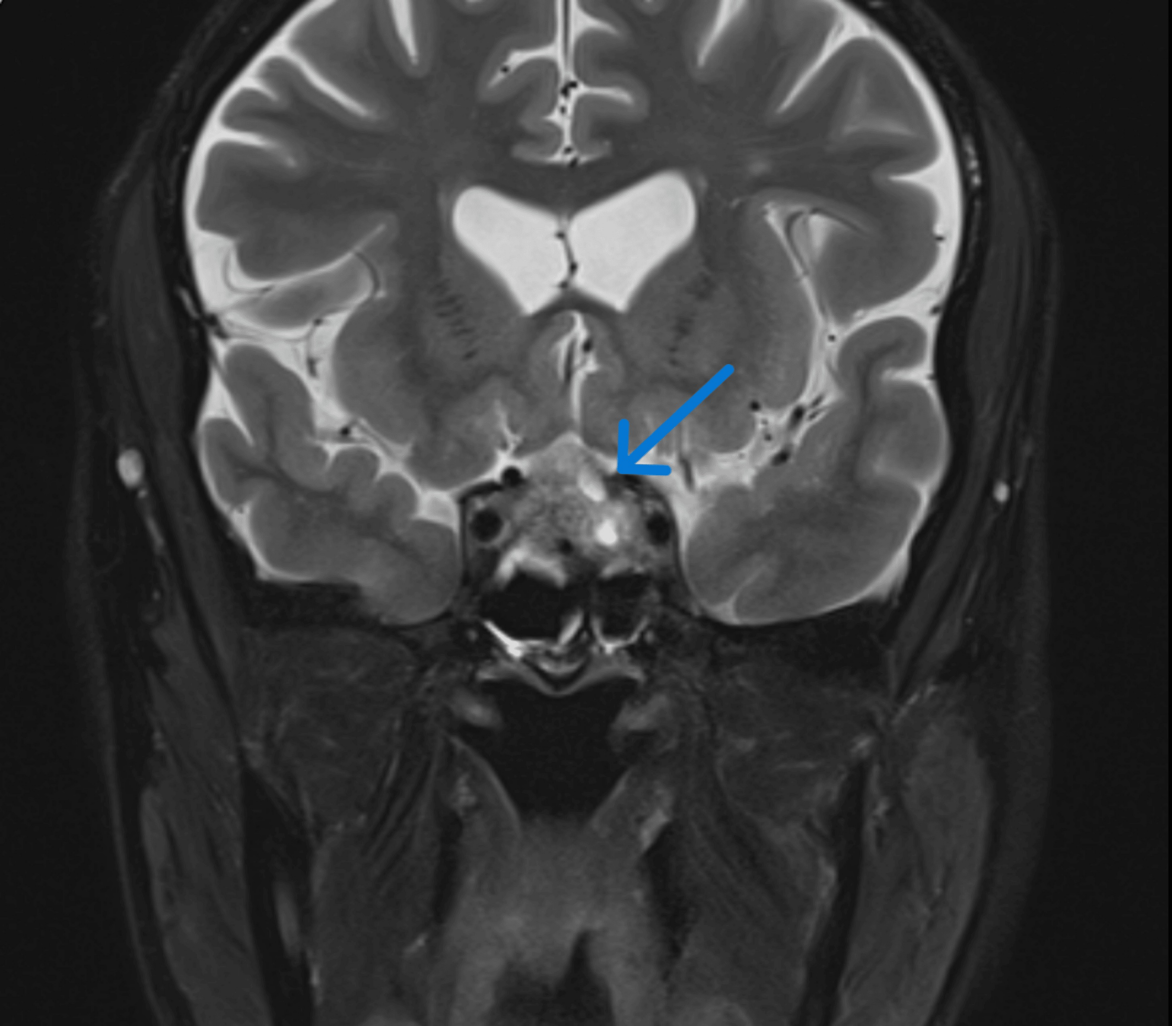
MRI (coronal view) showing pituitary apoplexy with compression of optic chiasm and partial obliteration of bilateral cavernous sinuses.
